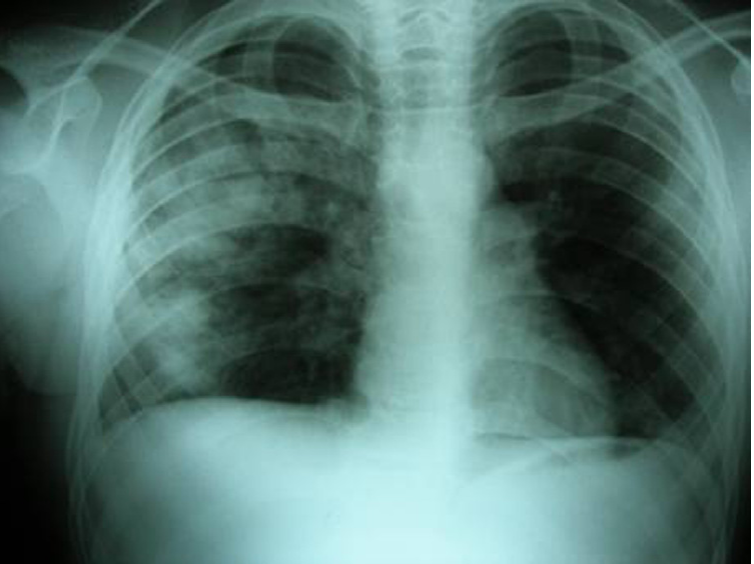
The direct x-ray image of the first patient on the 2^nd ^day of the trauma. Two cavitary lesions on the right and accompanying low-percentage pneumothorax are observed.
